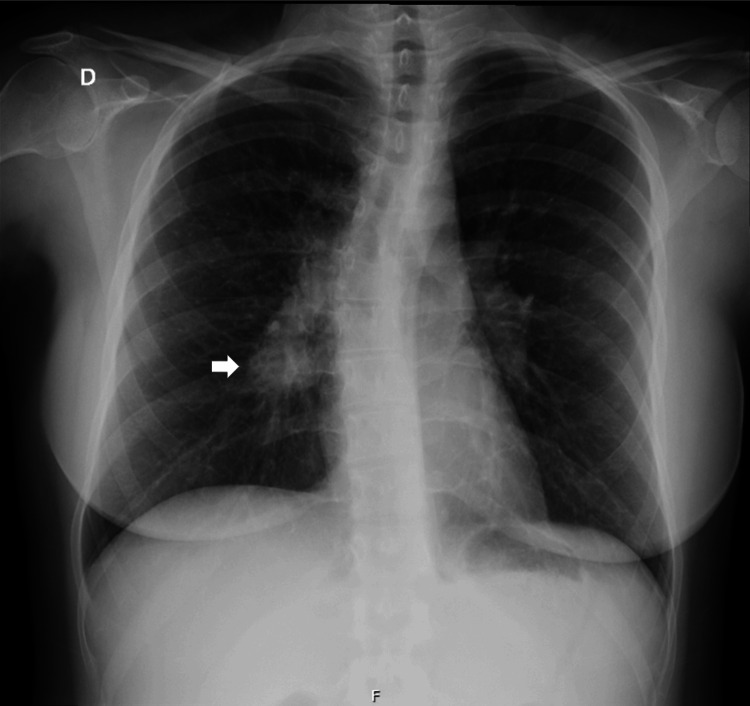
Posteroanterior chest X-ray. Performed on May 17, 2024, in the Emergency Department depicting right juxtahilar nodular hypotransparency (arrow).
